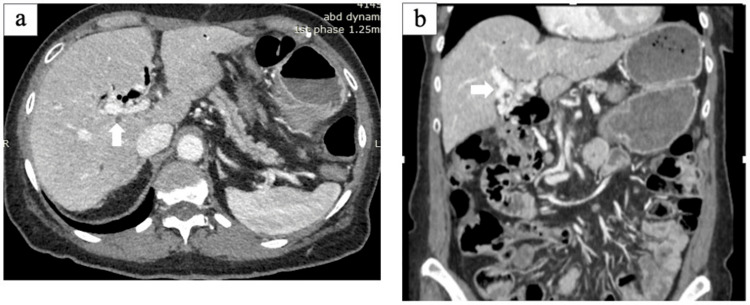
CT performed during gastrointestinal bleeding (a, b) Axial and coronal CT images show ectopic varices around the choledochojejunostomy (arrow)
